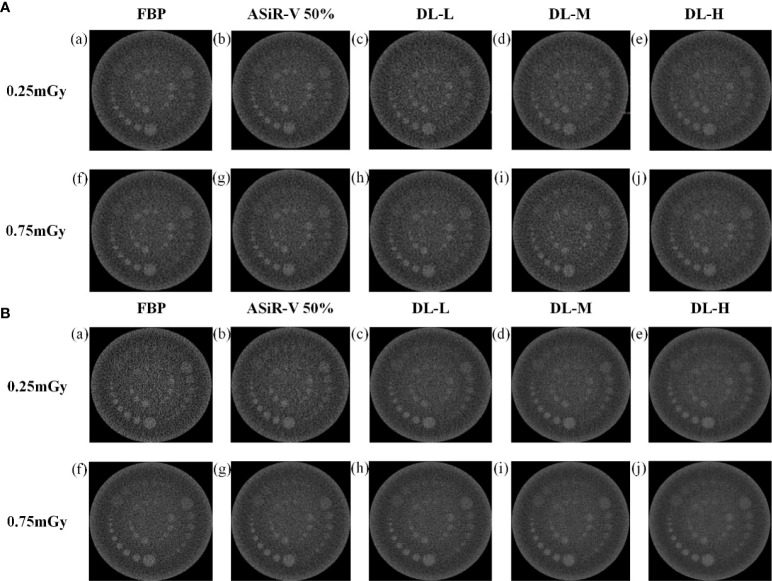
Low-contrast detectability images in helical mode reconstructed with FBP (a, f), ASiR-50% (b, g), and DLIR(L/M/H) (c, h; d, i; e, j) at 0.25mGy and 0.75mGy with a slice thickness of 1.25mm (A) and 5mm (B), respectively. CT, computed tomography; FBP, filtered back projection; ASiR-50%, adaptive statistical iterative reconstruction-veo 50%; DLIR(L/M/H), deep-learning image reconstruction, level low, medium, and high; mGy, milligray.
